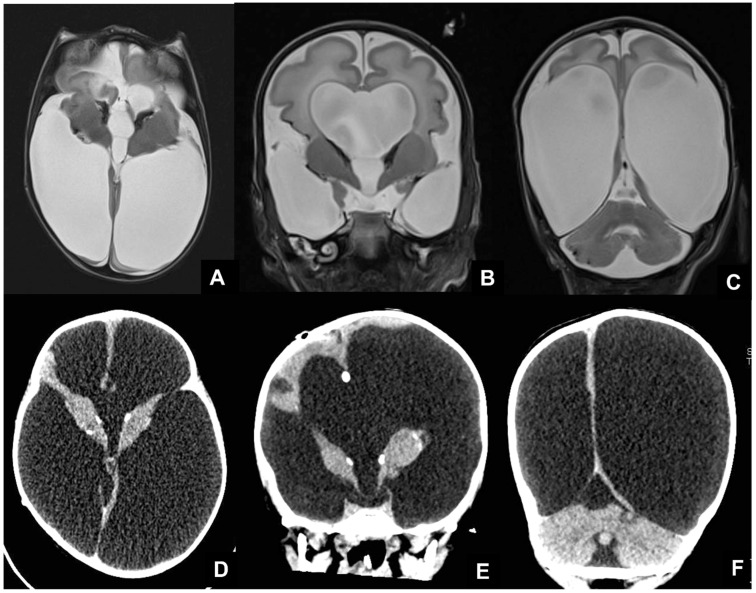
Pediatric patient affected by congenital CMV, undergoing MRI at four days of birth (A–C) and CT at six months of life (D–F), showing massive supratentorial ventricular dilatation and linear subependimal calcifications.
